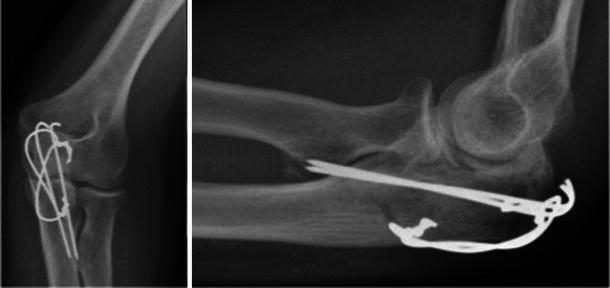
Radiographs at 2-month follow-up showed heterotopic ossification around the tips of the wires
